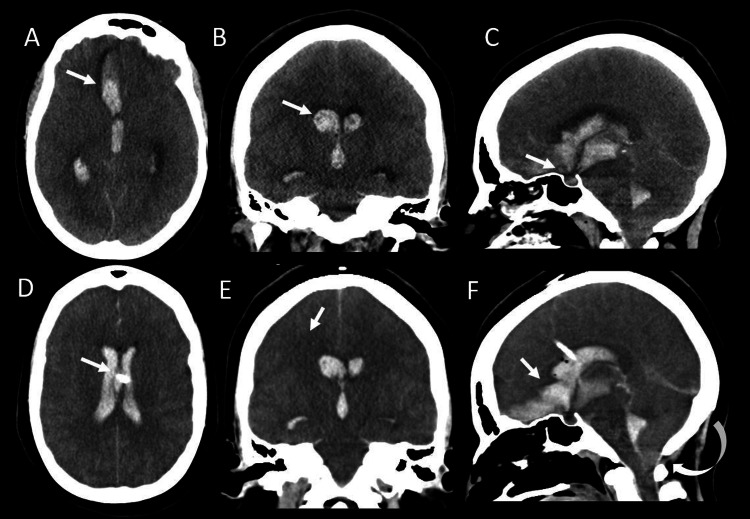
Nonenhanced computed tomography of the head Axial (A) nonenhanced CT with coronal (B) and sagittal (C) reformats demonstrates right inferior frontal lobe parenchymal hemorrhage (arrow in A) with large right more than left intraventricular hemorrhage (arrow in B) and mild subarachnoid hemorrhage (arrow in C). Right more than left ventriculomegaly. Follow-up CT head (D, E, F) without contrast demonstrates interval placement of a ventriculostomy catheter (arrow in D) and persistent diffuse narrowing of cerebral sulci with diffuse brain swelling. Early decreased gray-white matter differentiation, which is more conspicuous in the basal ganglia (arrow in E). Demonstrated right frontal parenchymal hemorrhage with large intraventricular and subarachnoid hemorrhage, which has enlarged intervals (arrow in F). There is early transtentorial and tonsillar herniation (curved arrow in F).
